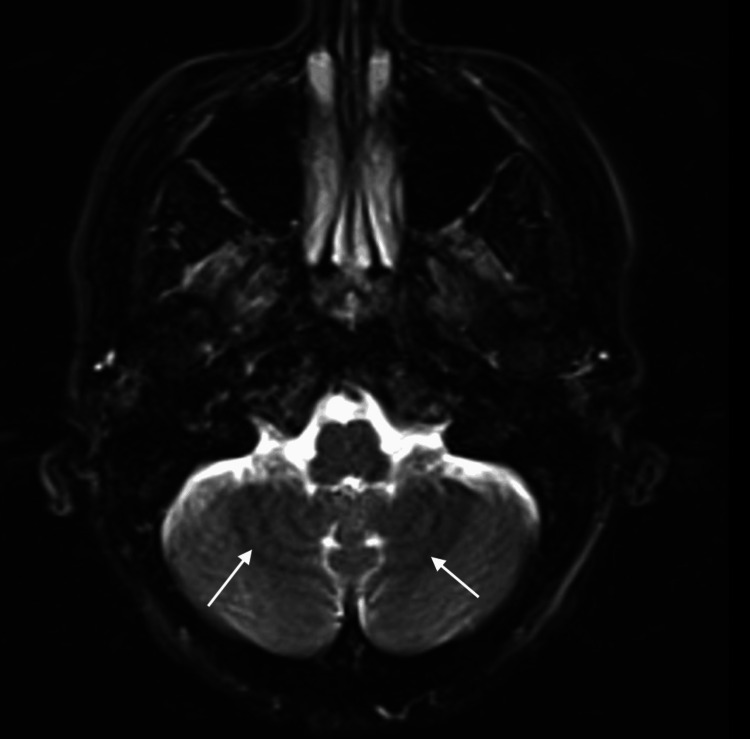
Case 1: MRI of the brain, T2-weighted image The hemosiderin deposition was not clearly visualized on conventional T2-weighted MRI images (arrowed).
